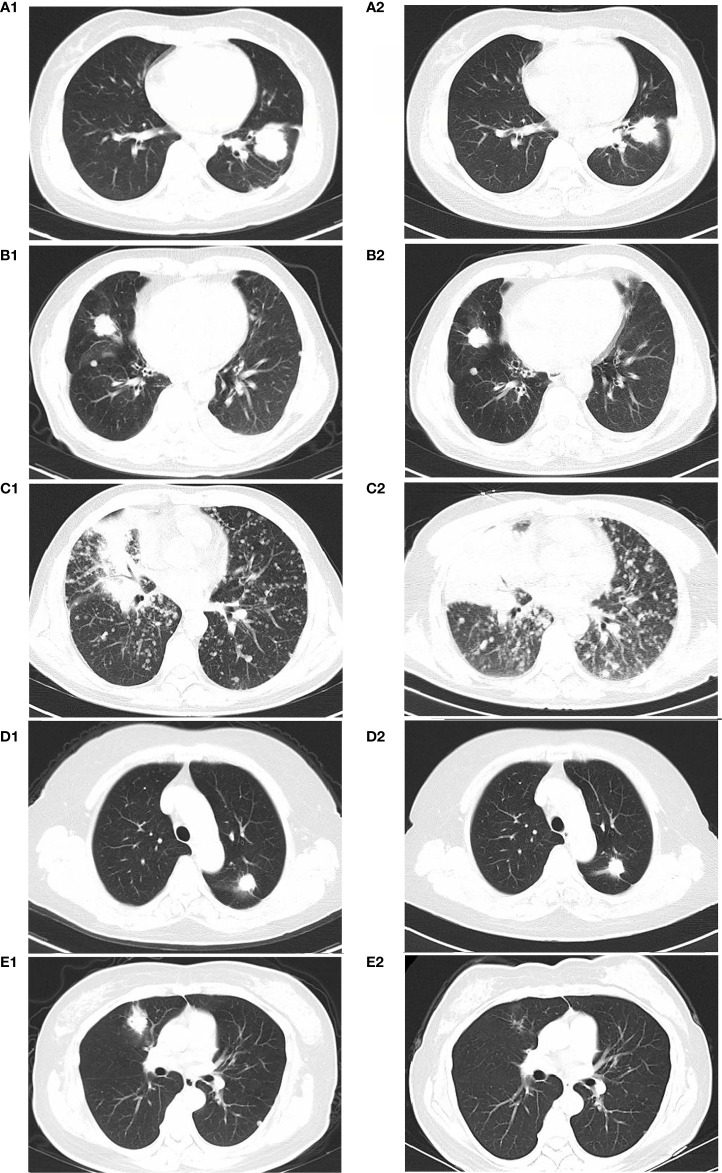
CT imaging of best disease response of patients: Case 1 at beginning of therapy (A1) and 4 months after receiving carboplatin+pemetrexed chemotherapy and sintilimab (A2) Case 2 at beginning of therapy (B1) and 5 months after receiving carboplatin+pemetrexed chemotherapy and sintilimab (B2) Case 3 at beginning of therapy (C1) and 2 months after receiving carboplatin+pemetrexed chemotherapy and pembrolizumab(C2) Case 4 at beginning of therapy (D1) and 10 months after receiving carboplatin+pemetrexed chemotherapy and pembrolizumab (D2) Case 5 at beginning of therapy (E1) and 6 months after treatment with carboplatin, pemetrexed, and pembrolizumab for 4 cycles followed by pembrolizumab maintenance (E2).
